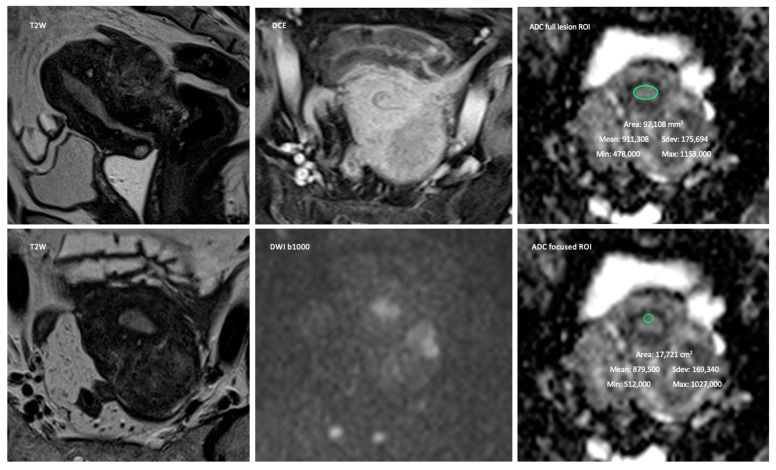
Seventy-year-old female with a leiomyoma staged at MRI as T1a and proven at pathology to be a T1a. Leiomyomas are one of the pitfalls for the determination of myometrial invasion by tumor. Depiction of the method used for the quantitative data collection using the two different ROI.
